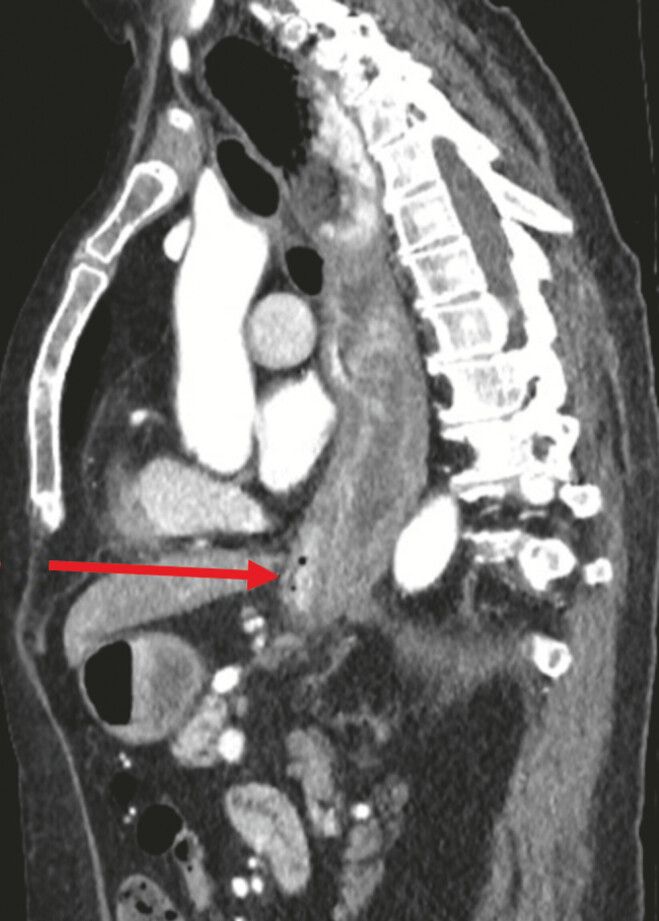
A sagittal CT scan with oral contrast showing no mediastinal leak with a dilated oesophagus (red arrow). CT, computed tomography.
